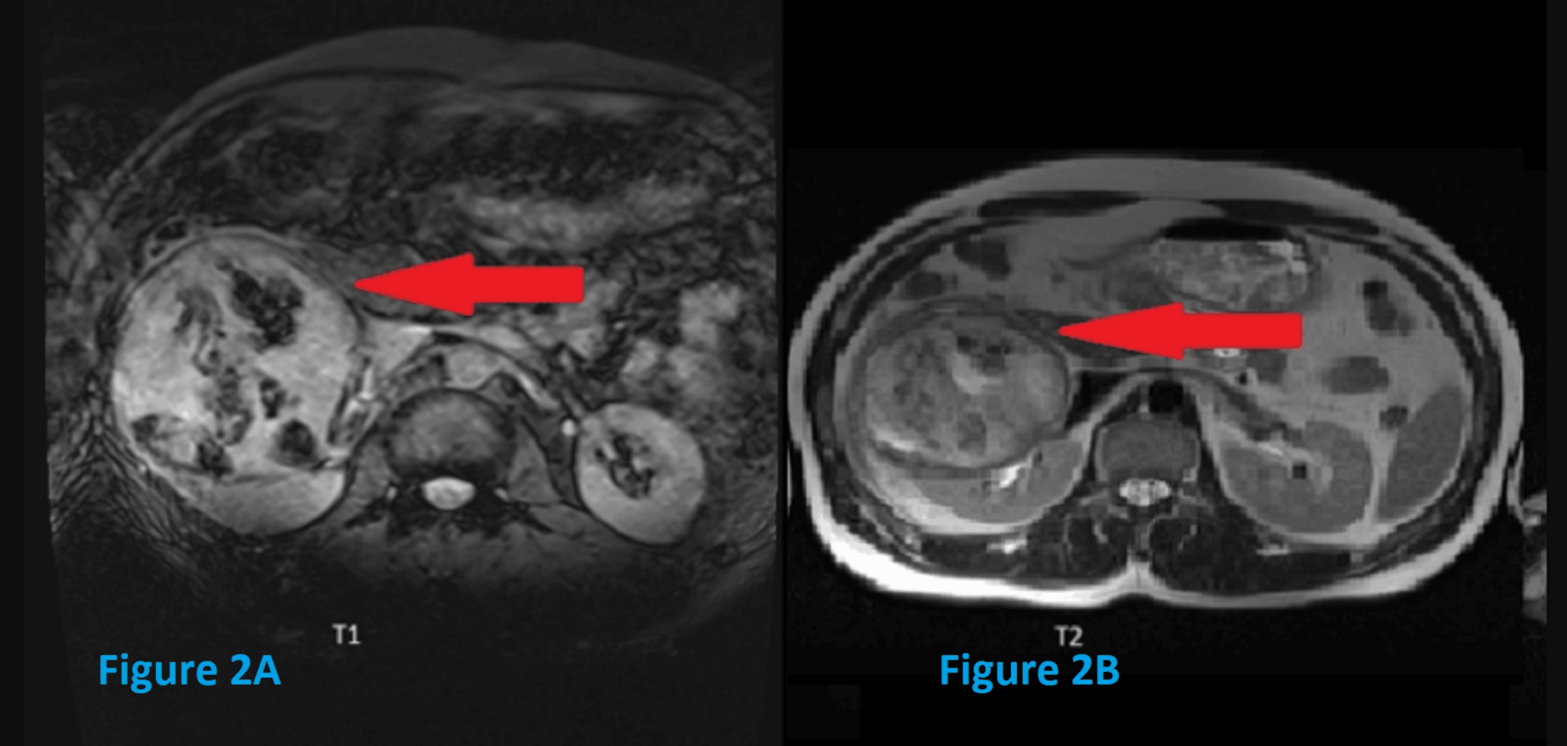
MRI images (A) A right renal lesion with multiple areas of GRE blooming, which appears hyperintense on T1 and (B) large, well-defined, heterogeneously hyperintense right renal mass on T2 weighted imaging.
